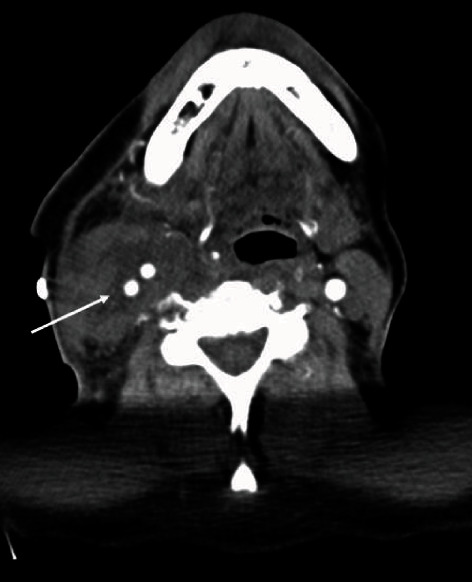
After 1 month from surgery, Computed Tomography (CT) scan shows good patency of the graft (arrow).
